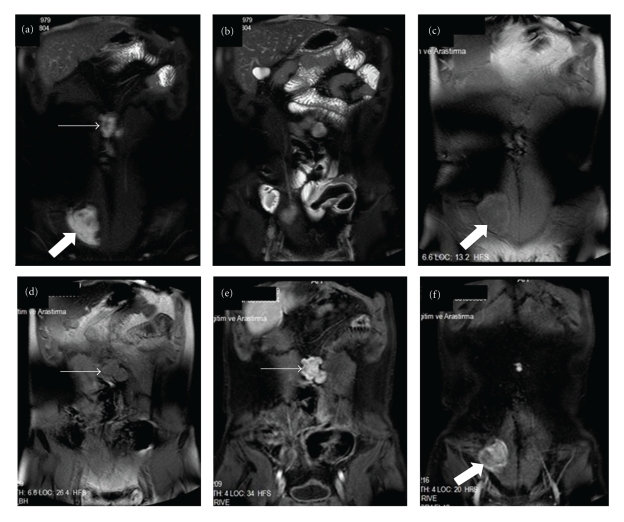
MR enterographic images of the patient 3. On coronal fat-saturated T2- weighted (W) HASTE (a), (b), T1W (c), (d), and contrast-material-enhanced T1W (e), (f), images show the desmoid tumors (thick arrows: inguinal desmoid tumor; thin arrows: mesenteric desmoid tumor). The relationship between contrast-material-enhanced mesenteric desmoid tumor and small-bowel loops is clearly seen on the MR images (arrow, (e)).
